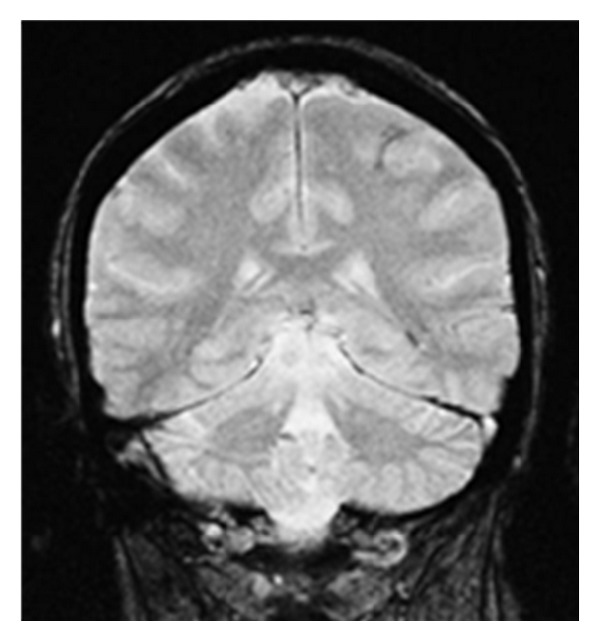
Third case of isolated central sulcus hemorrhage. Gradient echo MR images in a 50-year-old female with history of renal transplant and presenting with left leg weakness demonstrated signal loss within the left central sulcus consistent with an isolated hemorrhage. Associated nonspecific white matter changes were also seen. Angiographic work up was without any evidence of aneurysms or vascular malformations. Although the age of the patient and absence of other areas of chronic hemorrhage on MR imaging make this an atypical presentation, findings of an isolated central sulcus hemorrhage in a normotensive patient without other etiologies accounting for that hemorrhage would suggest a possible diagnosis of CAA.
